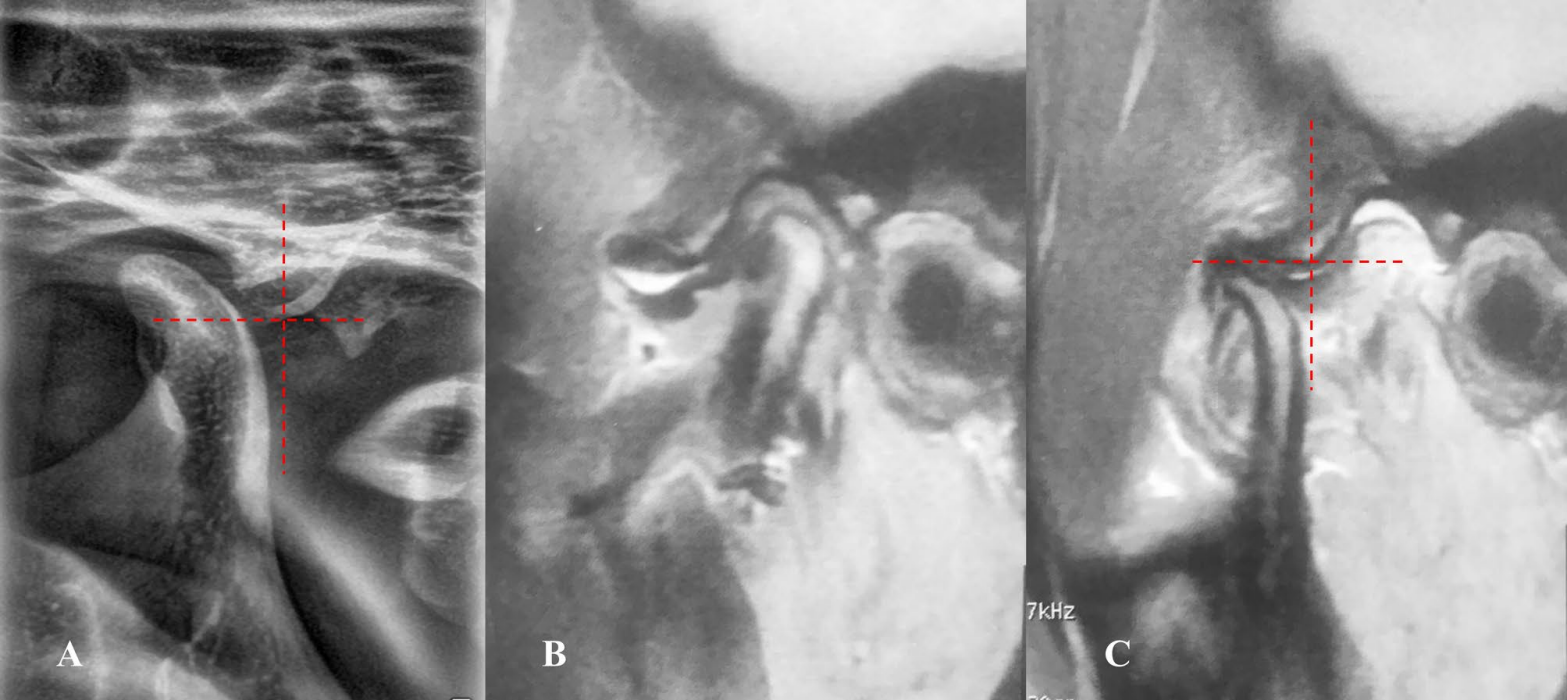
Lateral TMJ view represents Stage III-B (open lock) in open (A) mouth position, showing the condyle located anterior to the eminence but at a superior level to the highest point of the eminence. Oblique sagittal T2-weighted images (done after reduction of the open lock) represent Stage III-B, showing disk displacement in closed (B) mouth position and disk recapture in open (C) mouth position with joint effusion. The disk-condyle complex is located in front of the tip of the eminence or high up in front of the base of the eminence.
